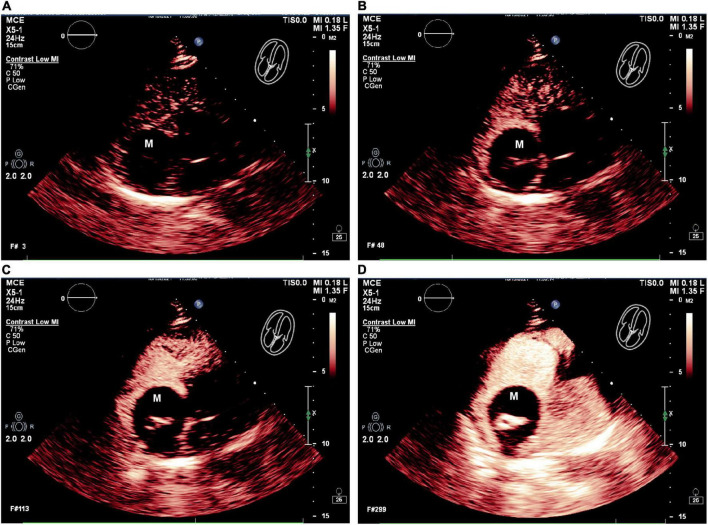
The RA mass was seen on contrast enhancement ultrasound (CEUS). (A–D) CEUS results showed that in different cardiac cycles, which the solid echo mass in the right atrium tumor revealed high contrast enhancement, and no contrast enhancement is evident in the surrounding anechoic area. M = (intracardiac mass).
